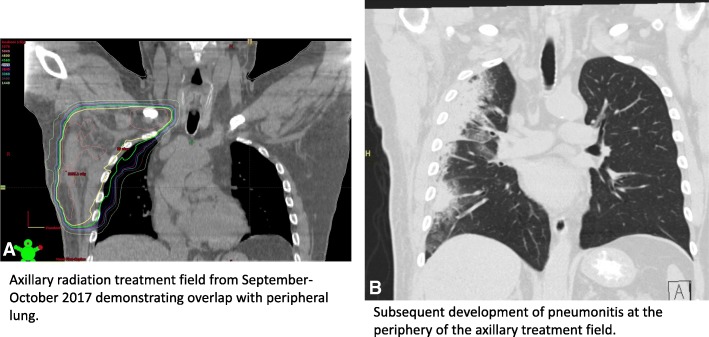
a (left) Axillary radiation treatment plan with radiation isodose curves overlaid demonstrates peripheral overlap with the lung b (right) Subsequent CT of the chest performed after starting ICB demonstrates peripheral consolidation and opacities at the periphery of the axillary treatment field
